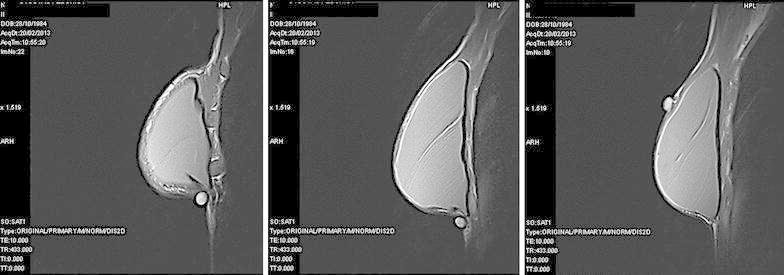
MRI images, February 2013*. Left* the first nodule (suggestive of granuloma) in the lower-inner quadrant. *Center* the nodule at the inframammary fold, at a distance of 2 cm from the first nodule. *Right* the nodule in the upper-outer quadrant (suggestive of breast cancer recurrence)
